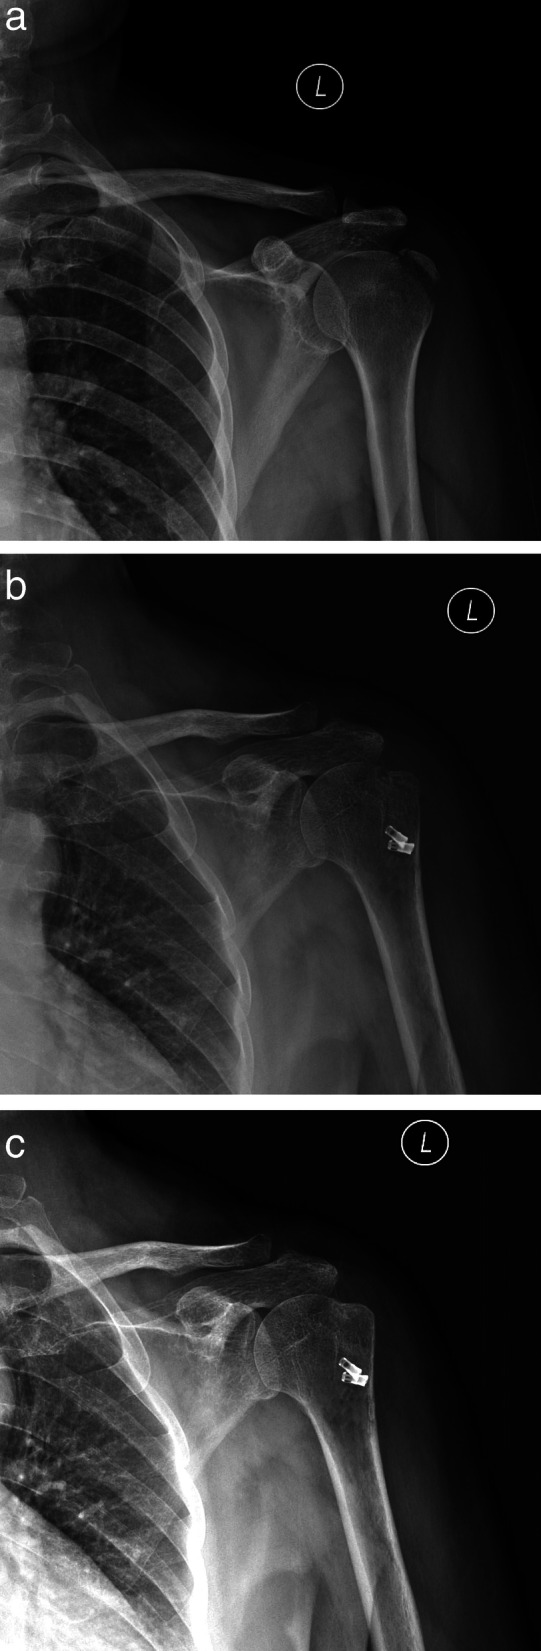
a Pre-operative X-ray. b Postoperative X-ray examination. This shows that the fracture reduction was good. c Fracture healing
